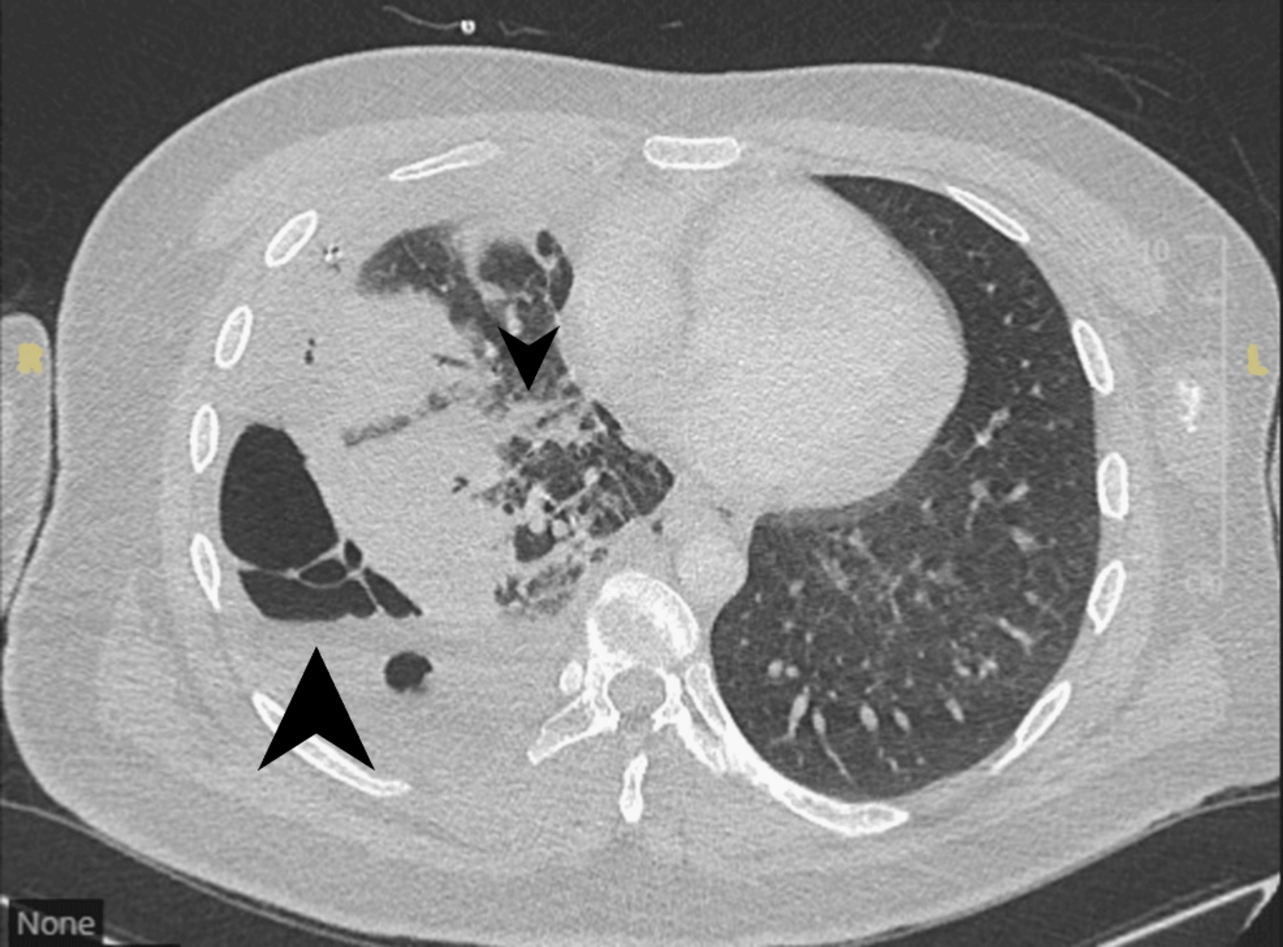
Axial CT thorax at the level of the lower lobes demonstrates a right-sided multiloculated hydropneumothorax with multiple septations. There is clear communication with a cavitating lesion in the right lower lobe (arrowhead), concerning for a bronchopleural fistula. CT: computed tomography
